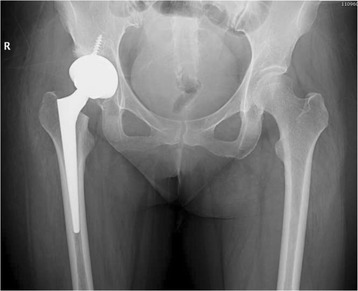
Immediate postoperative anteroposterior radiographs demonstrating correct placement of the implant. Press-fit of the stem was achieved
